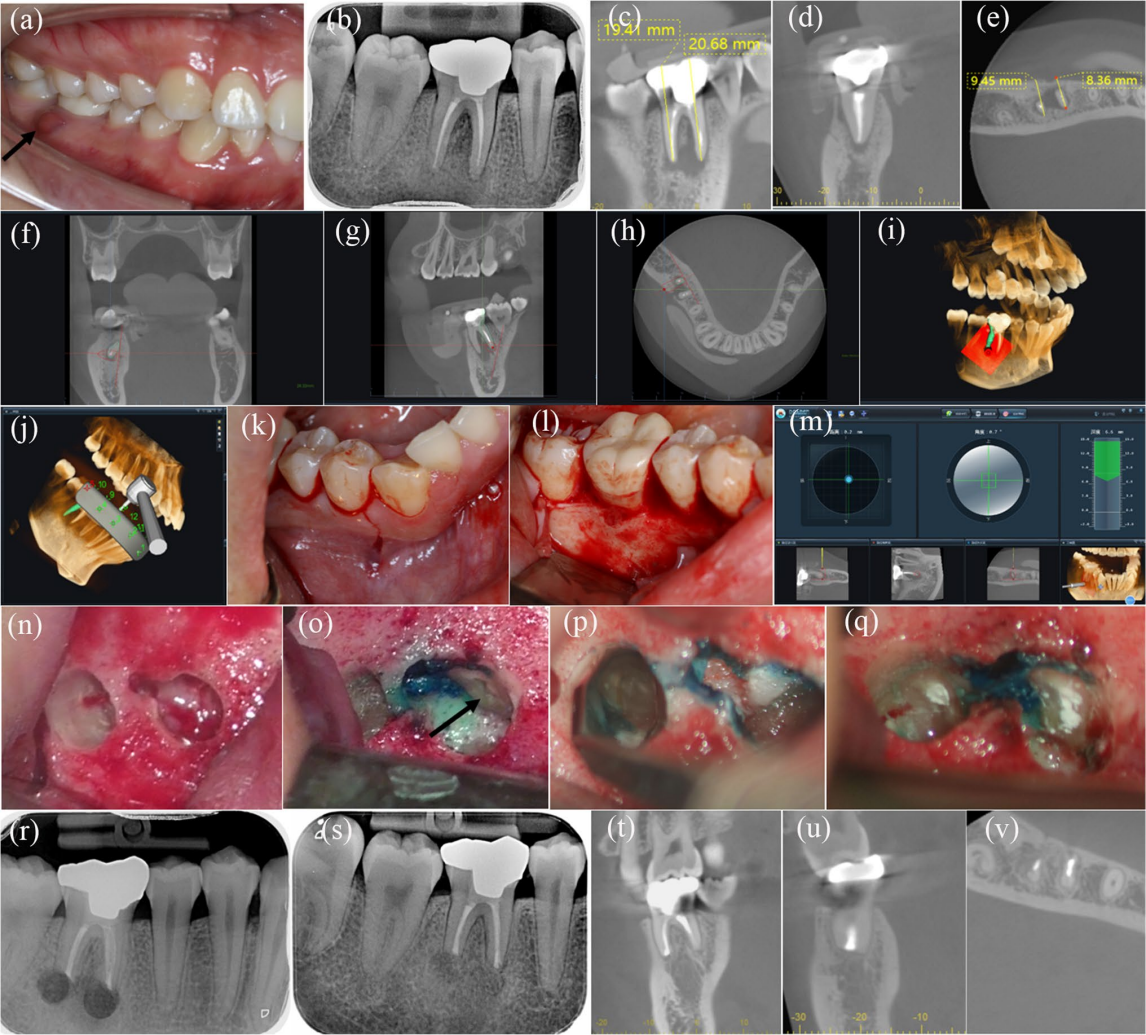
Typical posterior dynamic navigation (DN)–aided endodontic microsurgery case in tooth 46. a Preoperative photograph; the black arrow indicates the sinus tract. b Preoperative radiograph. c–e Preoperative sagittal, coronal, and axial cone-beam computed tomography (CBCT) sections and measurement. f–h Surgical path planning in DN software of coronal, axial, and sagittal view, respectively. i Three-dimensional view of surgical path planning in DN software. j Registration. k Incision. l Flap elevation. m Real-time views in the screen of DN system. n After guided osteotomies and root-end resections. o, p Retrograde preparation of mesial and distal root, respectively; black arrows indicate the root resection plane. q Retrograde filling. r Immediate postoperative radiograph. s One-year follow-up radiograph. t–v One-year follow-up CBCT of sagittal, coronal, and axial view
